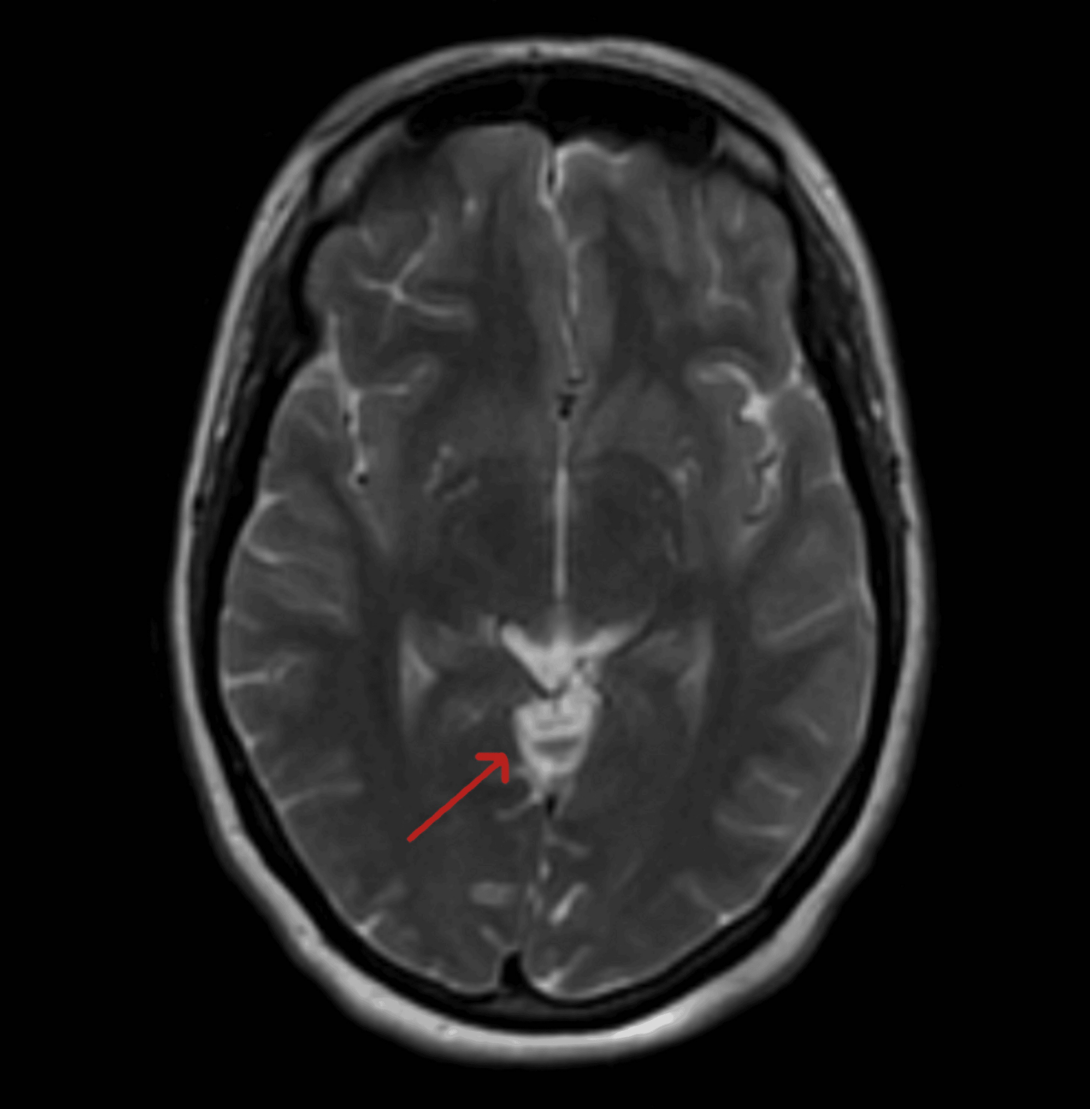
Axial T2-weighted MRI confirming a CSF-intensity lesion in the quadrigeminal cistern with typical signal characteristics of an arachnoid cyst and without associated parenchymal edema (arrow). CSF: cerebrospinal fluid
